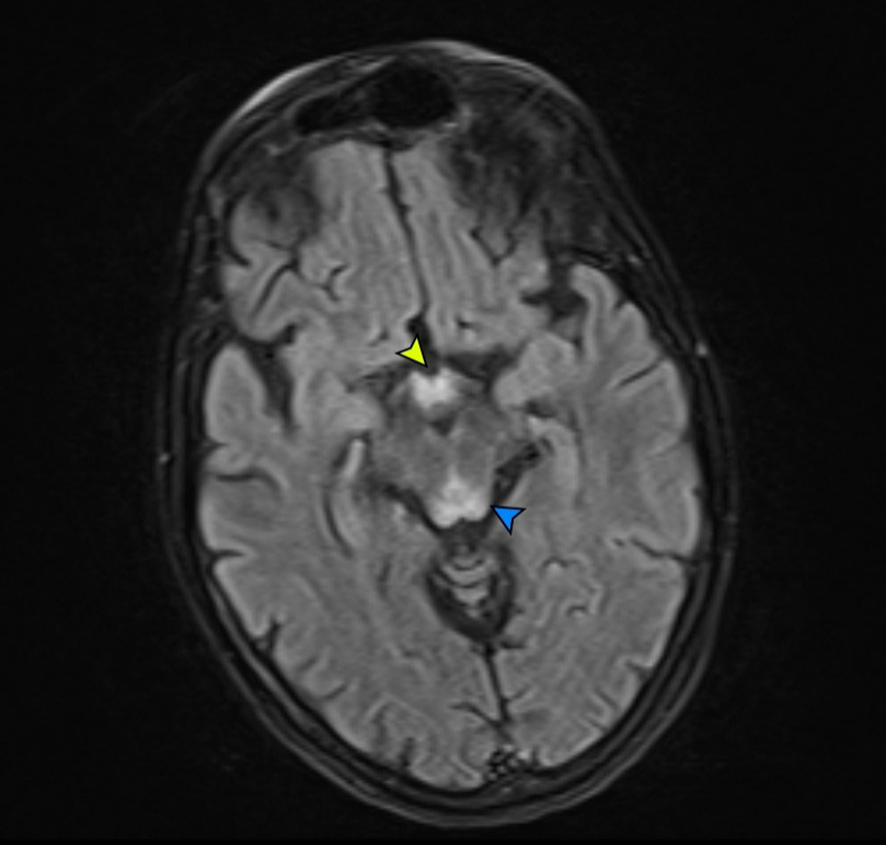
Mamillary bodies involvement. FLAIR axial image at admission, showing increased signal in the mammillary bodies (yellow arrow) and the posterior midbrain (blue arrow).
